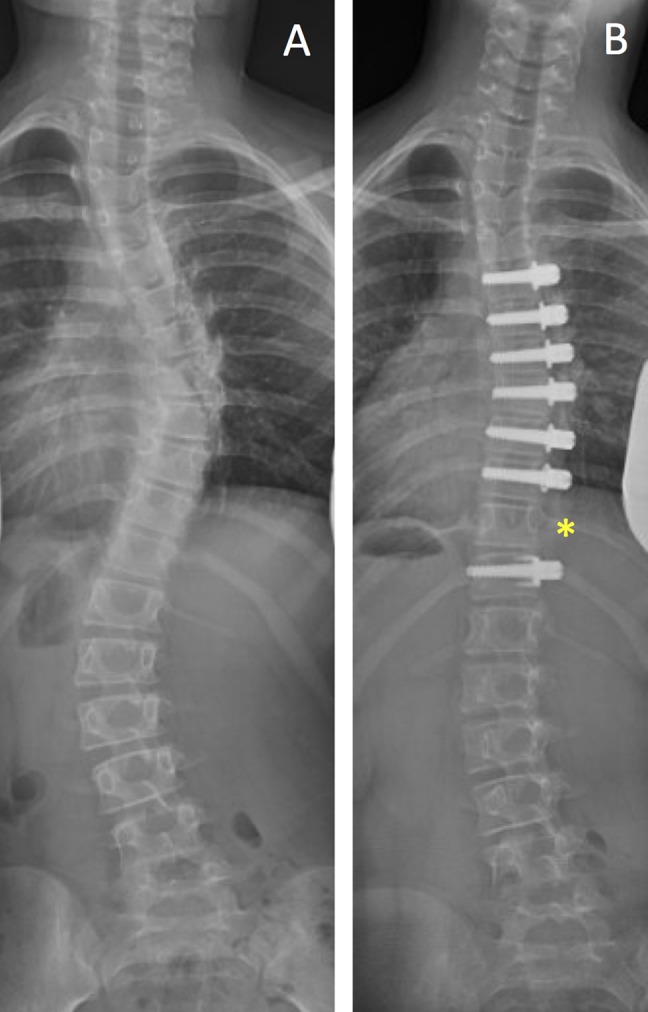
Radiograph showing a 9-year-old premenarcheal girl with progressive thoracic more than lumbar scoliosis who (A) underwent a right T5-T11 anterior spine instrumentation. No implant was placed at T11 (asterix) because a preoperative CT angiogram demonstrated the artery of Adamkiewicz at the right T11. In this patient, a second artery of Adamkiewicz was found on the left at L2. There were no neuromonitoring changes intraoperatively and no neural deficits postoperatively.
